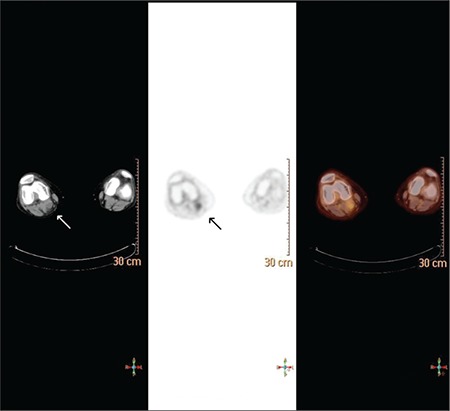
Axial slices of F-18 FDG PET/CT study showing an increased focal uptake behind patient’s right knee on PET scan (SUVmax 3.2) (black arrow). A fluid density on CT scan in the same location compatible with Baker’s Cyst (white arrow)
